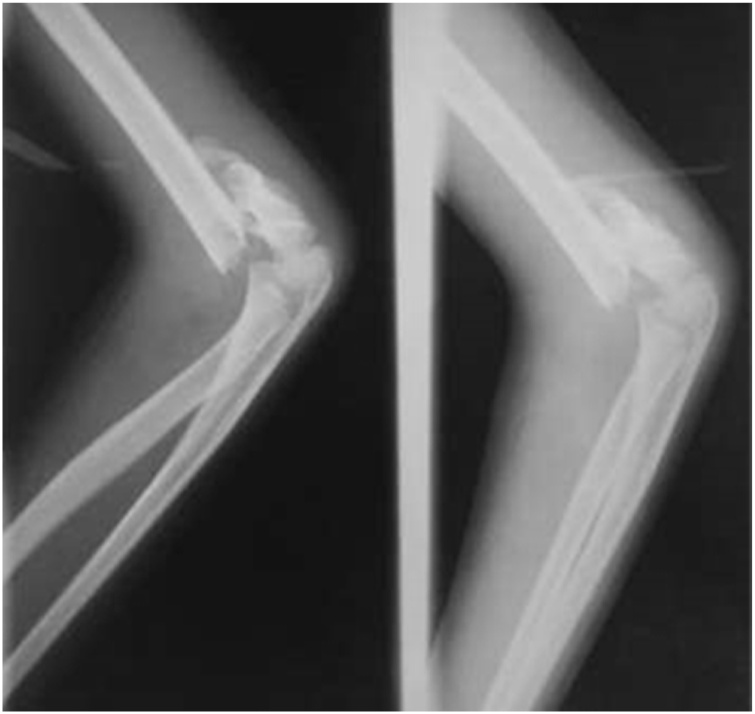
Radiographs were showing supracondylar humerus fracture (Gartland type III).
